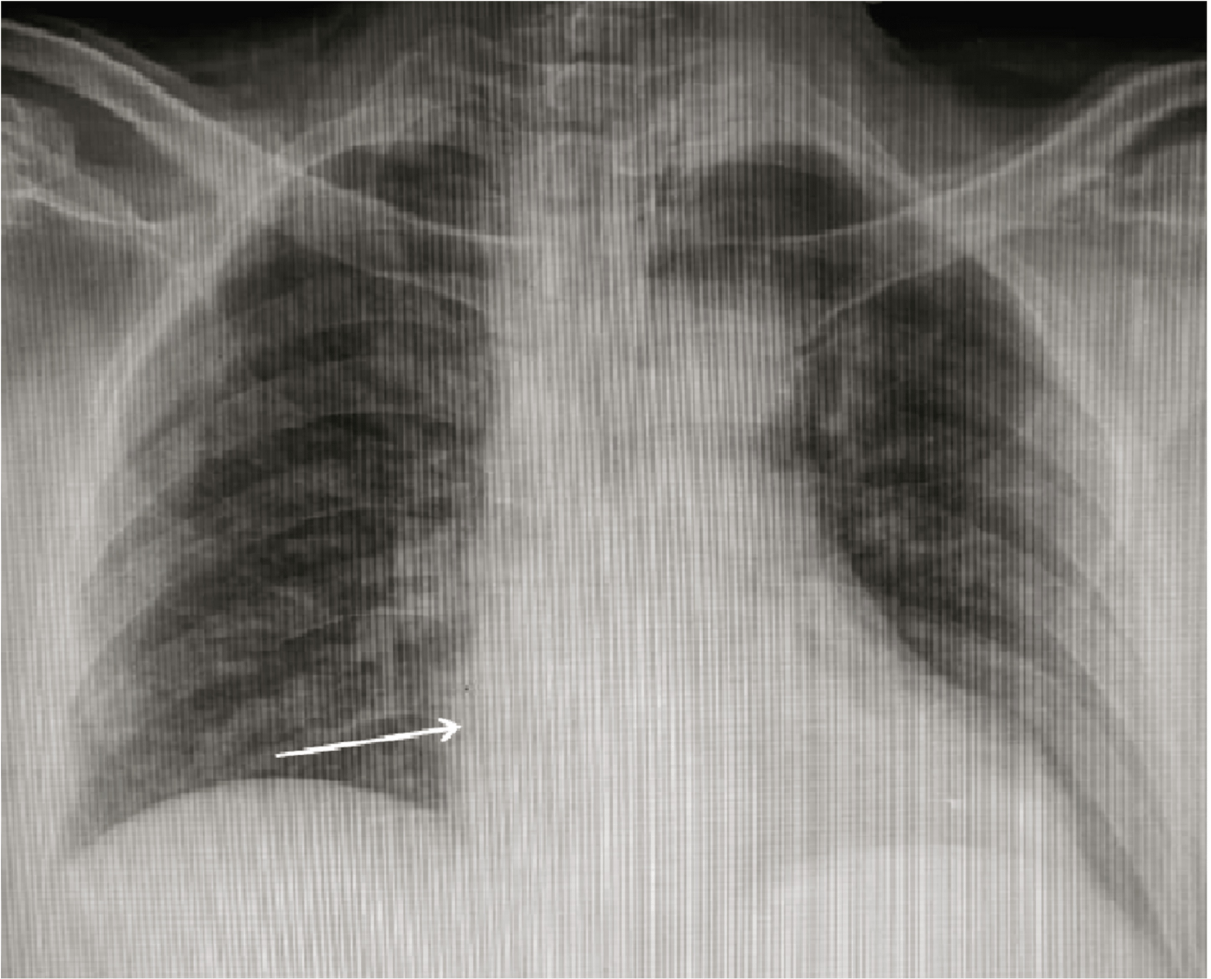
Chest X-ray anterio-posterior view suggestive of apparent cardiomegaly
